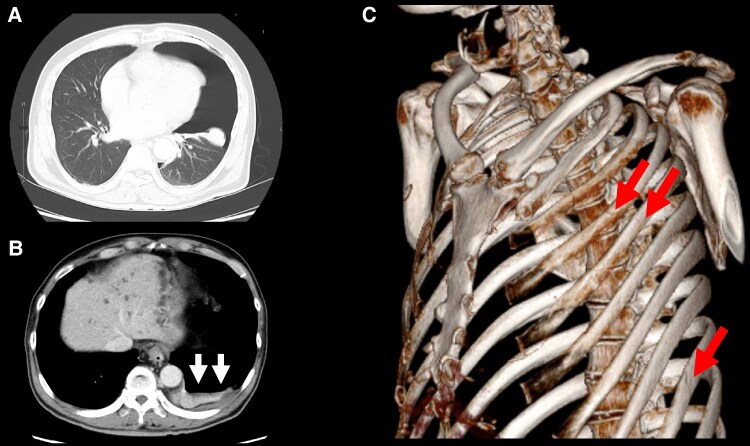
Chest computed tomographic images of a 73-year-old man. Chest computed tomography exhibited a collapsed left lung parenchyma (*A*) concomitant with blood components in the thoracic cavity (*B*, arrows), indicating haemopneumothorax. The three-dimensional image exhibited multiple rib fractures across the left fourth, fifth, and ninth ribs (*C*, arrows).
